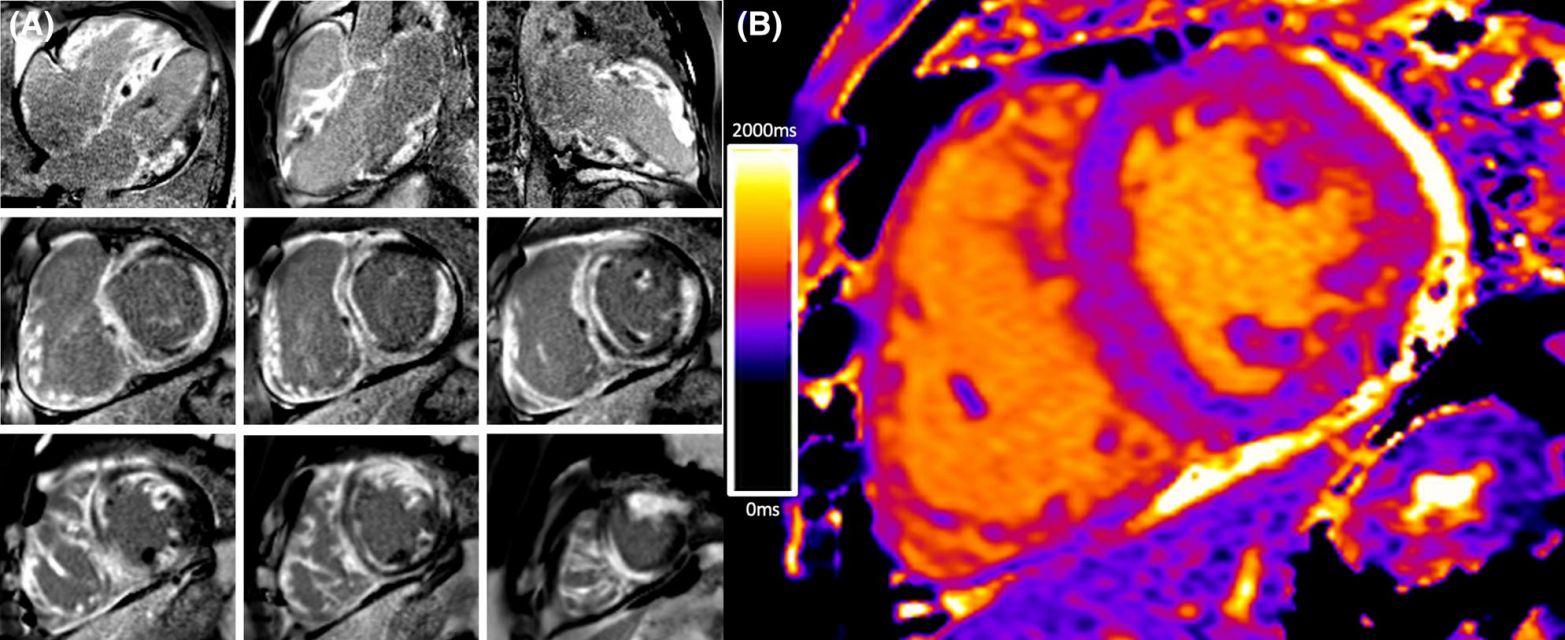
Cardiac magnetic resonance imaging. (A) Extensive biventricular delayed enhancement involving the right ventricle trabeculae. “Zebra-like” enhancement of the interventricular septum is often seen in cardiac amyloidosis. (B) Increased native myocardial T1 values in the mid-lateral wall
